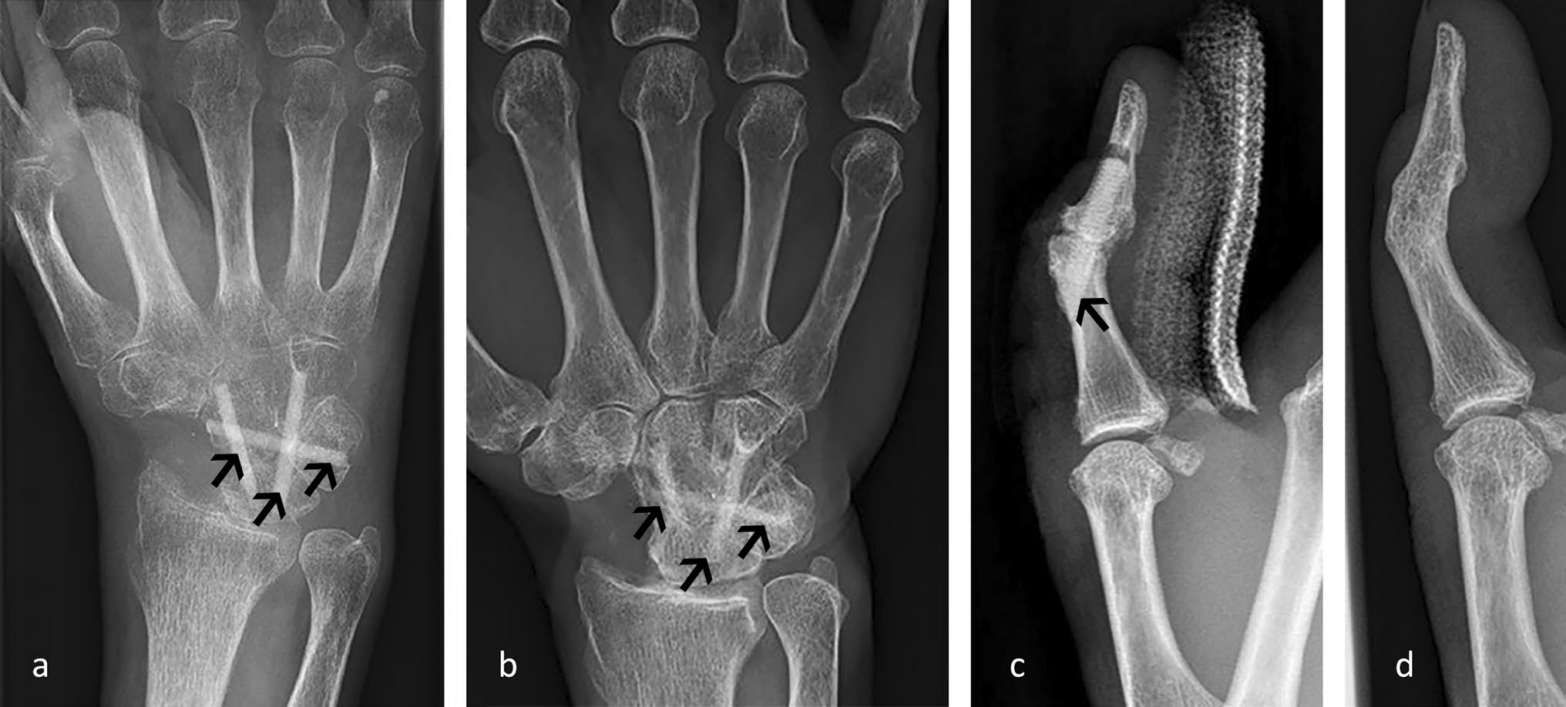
Examples of the application of the allogeneic screw in hand surgery. Postoperative radiographs showing the transplant used for a four-corner fusion a 10 weeks after surgery, b 20 months after surgery. Postoperative radiographs of an IP arthrodesis of the thumb c on the day of surgery, d 12 months after surgery. Transplants are marked by arrows
